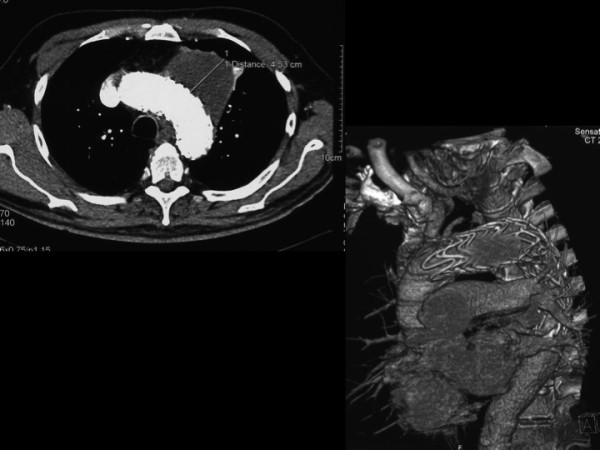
CT control after second operation: correct position of the graft and no leaks.
